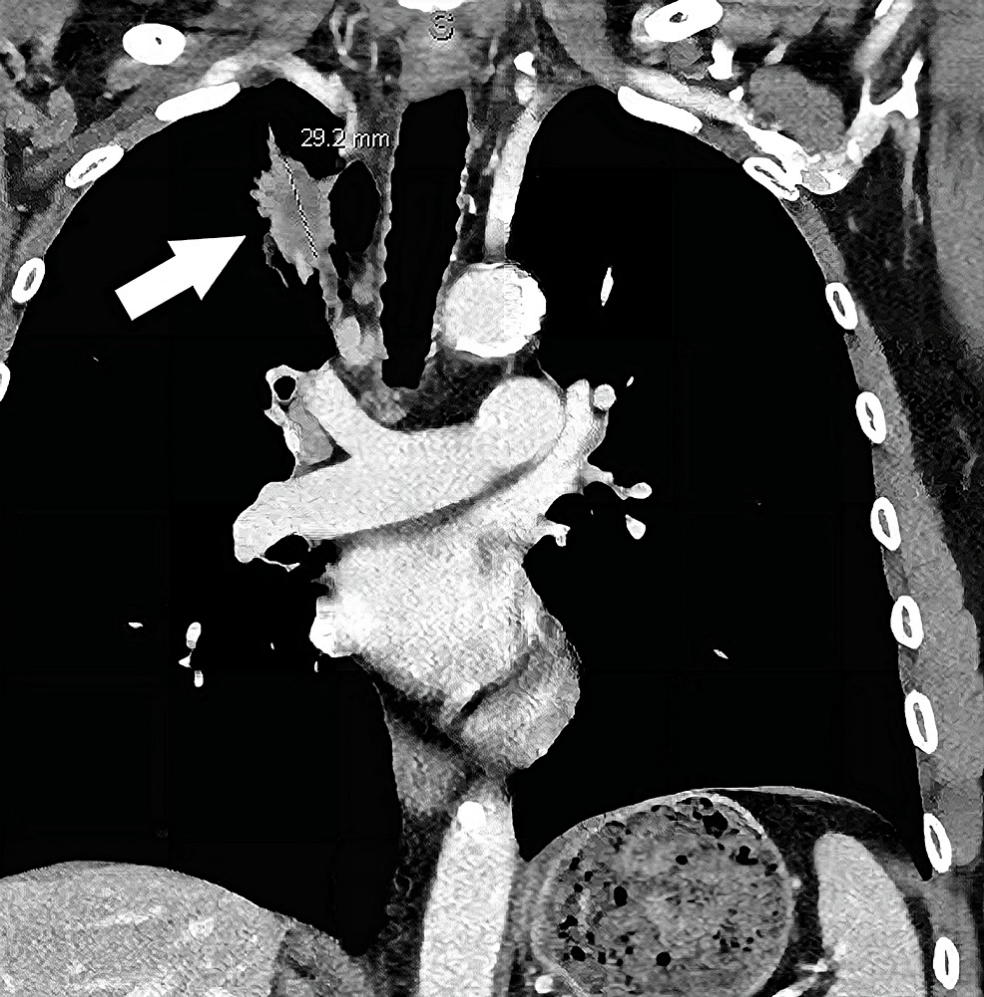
CT Chest at the Time of Diagnosis Shown here is the original imaging revealing a right upper lobe spiculated pulmonary lesion measuring 29.2 mm in diameter. This imaging was obtained after concerns for a primary lung tumor arose and was utilized in diagnosing the patient as the imaging confirmed the suspicion.
